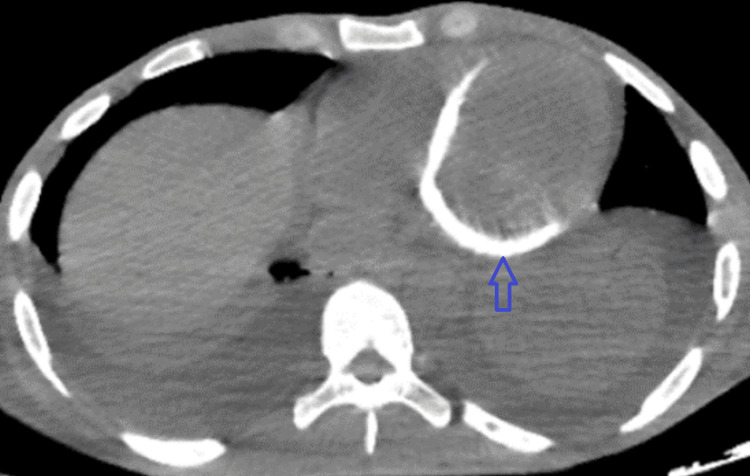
Noncontrast-enhanced CT scan of the chest on day 29 with the axial view showing extensive myocardial calcifications of the left ventricular wall associated with bilateral pulmonary pleural effusion. Blue arrow: myocardial calcifications of the left ventricle
